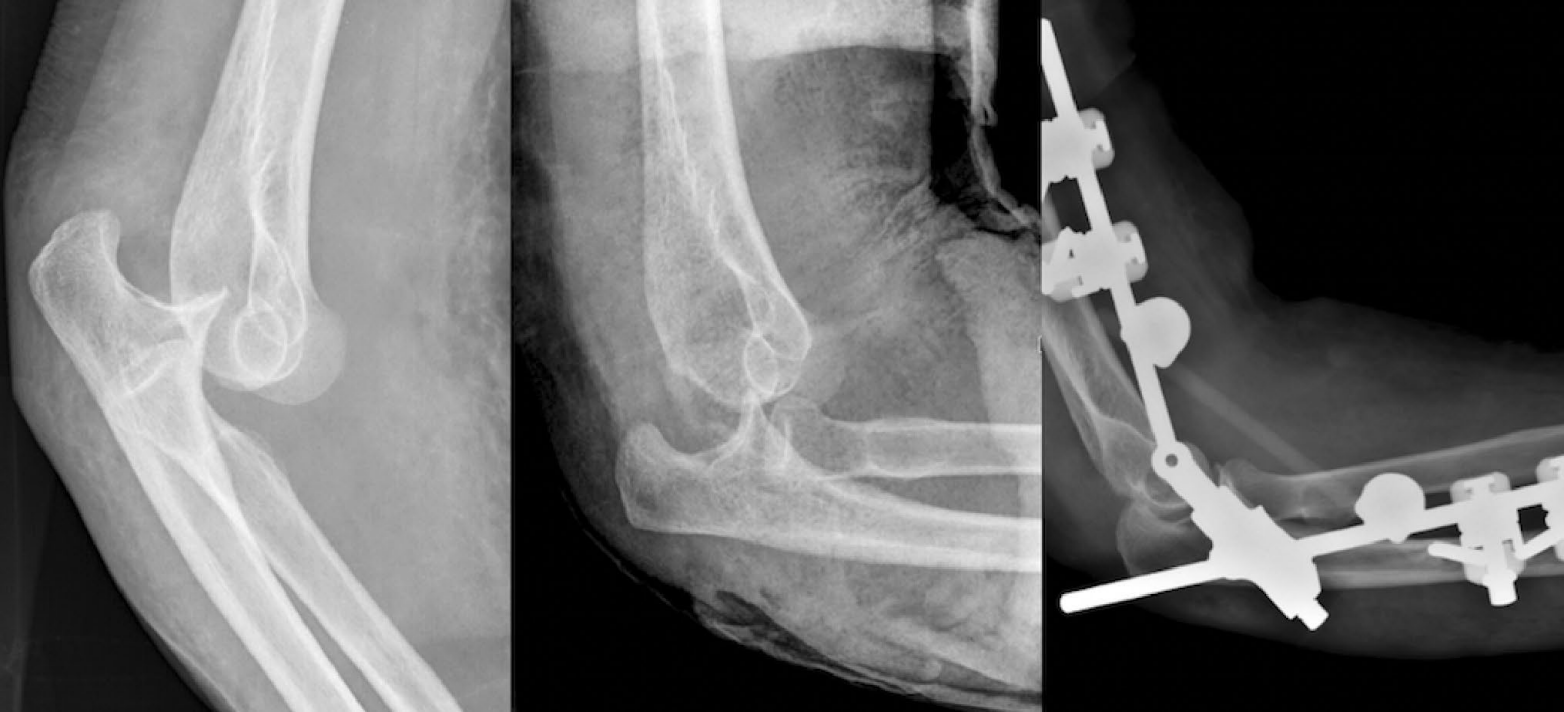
Lateral radiographs of unstable simple elbow dislocation. a Posterior elbow dislocation. b Subluxated joint after closed reduction. c Ligament repair with suture anchor and protective fixation with DJD hinged external fixator
